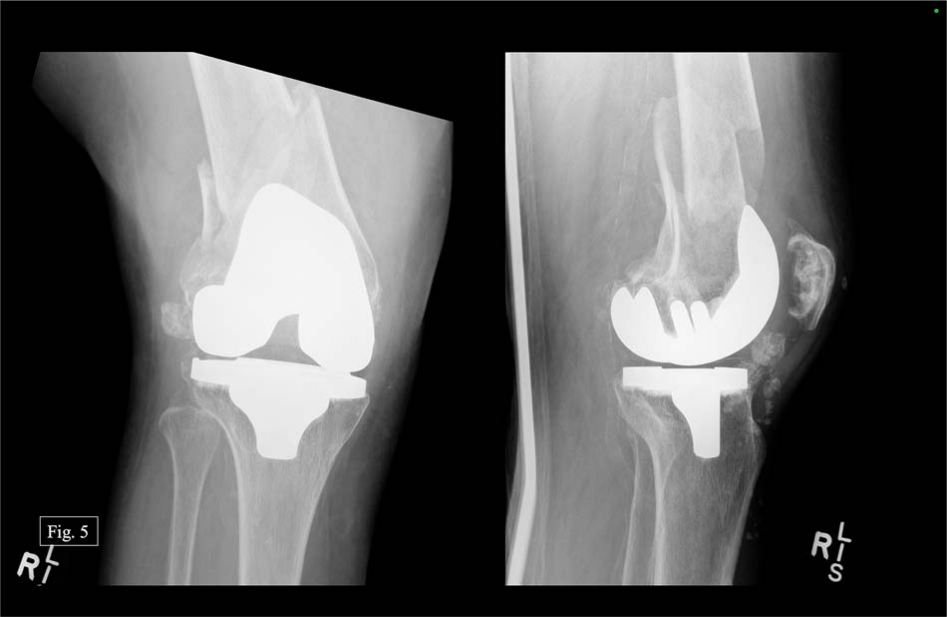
Right, acute distal periprosthetic femur fracture X-rays.
